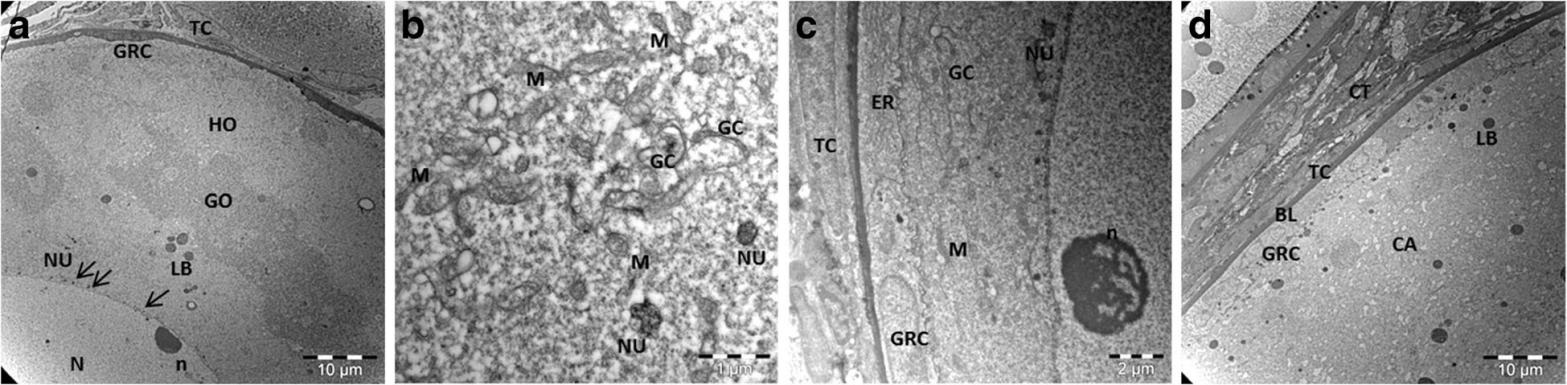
Ultrastructure of Atlantic salmon oocytes. a Stage 2 oocytes with granular ooplasm/Balbiani body. Black arrows indicate accumulations of nuage close to nuclear envelope and numerous nucleoli. b An area with aggregates of nuage and numerous mitochondria. c Ooplasm of stage 2 oocytes with endoplasmic reticulum and Golgi complex near the nuclear membrane. d Stage 4 oocyte surrounded with follicular cells. BL-basal membrane; CA-cortical alveoli; CT-connective tissue; ER-endoplasmic reticulum; GC-Golgi complex; GO-granular ooplasm; GRC-granulosa cell; HO- homogeneous ooplasm; LB-lipid body; M-mitochondria; NU-nuage; N-nucleus; n-nucleolus; TC-theca cell
